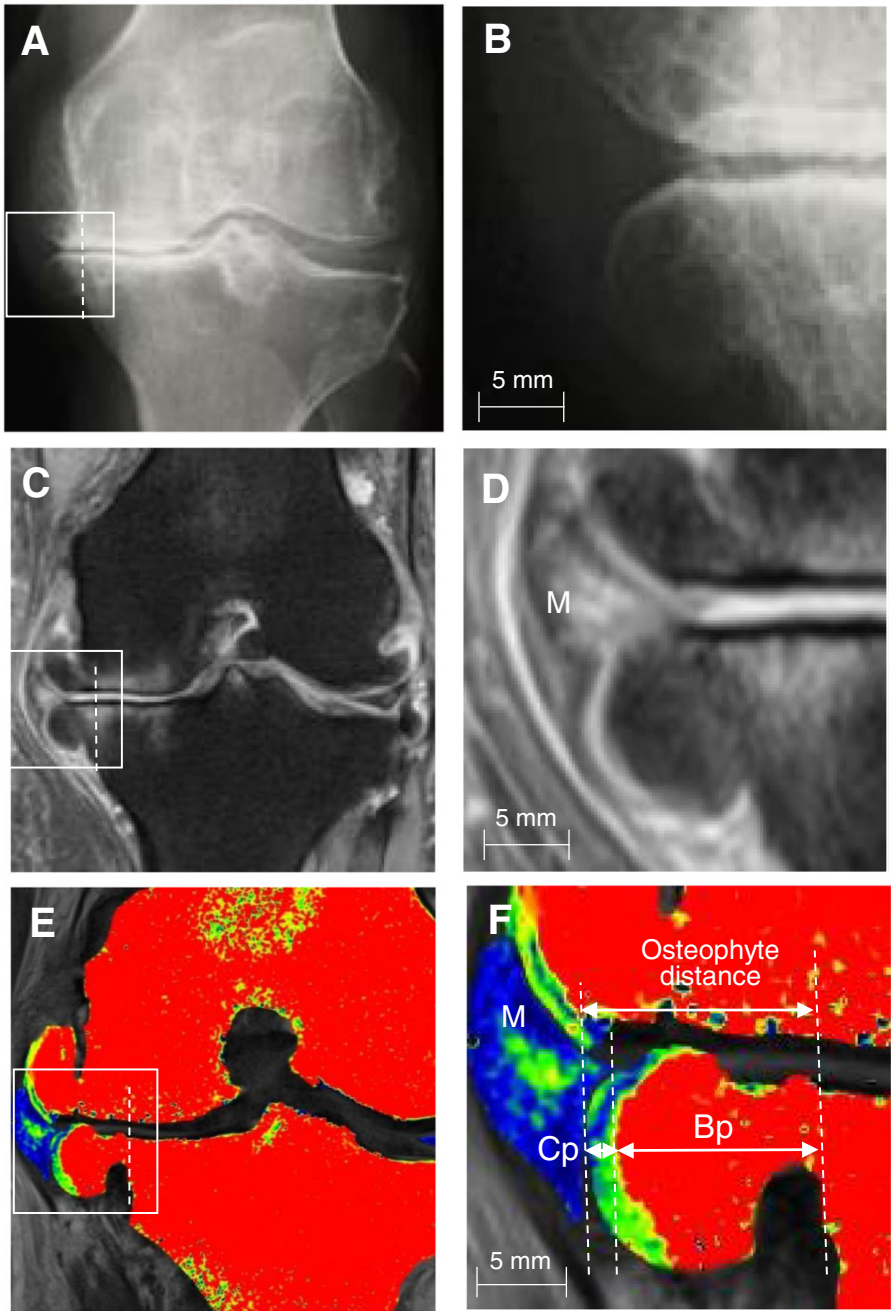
Findings of medial tibial osteophytes by radiography, proton density-weighted magnetic resonance imaging (MRI), and T2 mapping MRI. a Representative radiograph of the end-stage knee osteoarthritis joint. b High-power view of the boxed area in (a). c Proton density-weighted MRI with fat suppression of the same knee joint shown in (a). d High-power view of the boxed area in (c). e T2 mapping MRI of the same knee joint shown in (a). f High-power view of the boxed area in (e). *Bp* bone part of osteophyte, *Cp* cartilage part of osteophyte, *M* medial meniscus
